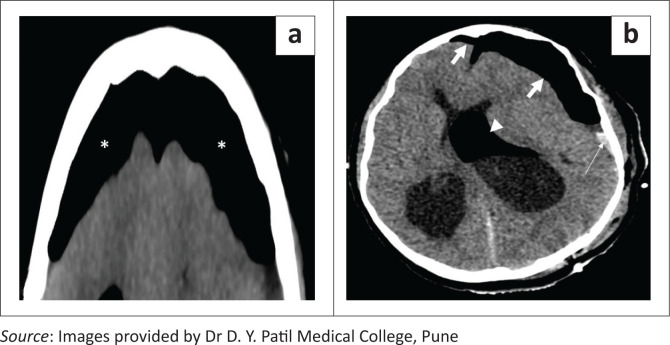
Axial soft tissue window CT image (a) shows tension pneumocephalus in both frontal regions (asterisks) causing mass effect on the underlying brain parenchyma with a heaped-up appearance of the anterior tips of the bilateral frontal lobes (Mount Fuji sign). Axial soft tissue window CT image (b) in a different patient shows asymmetric tension pneumocephalus (thick white arrows) in both frontal regions (left > right), causing mass effect on the underlying brain parenchyma and subfalcine herniation towards right side. There is an associated subdural hemorrhagic collection (thin white arrow) present on the left side and postoperative air in the left lateral ventricle (arrowhead).
